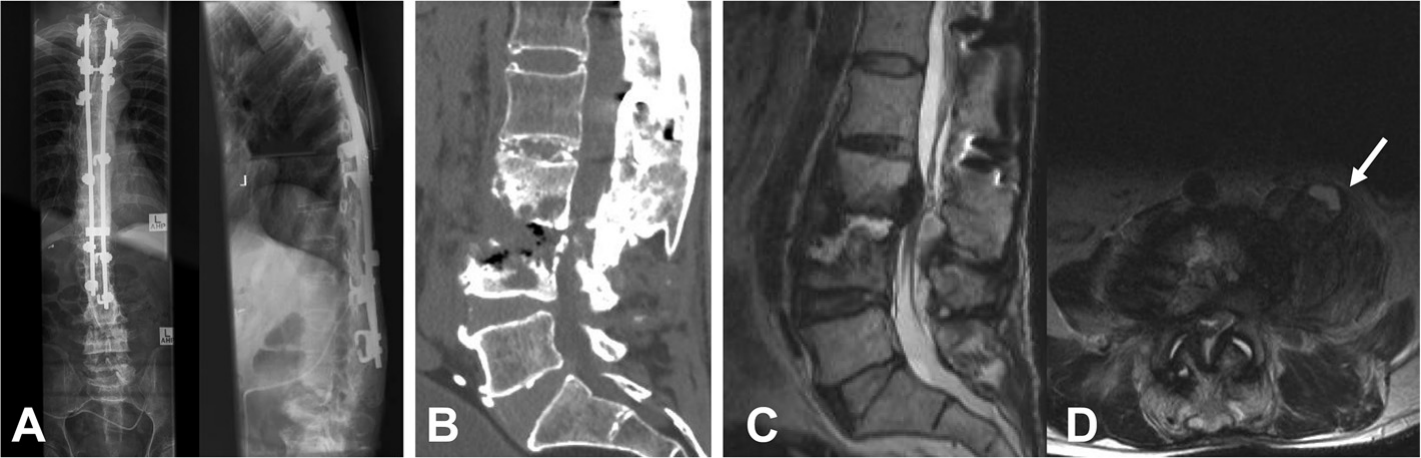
Plain radiographs, computed tomography and magnetic resonance imaging showing bone destruction and deformity consistent with Charcot spine below previous thoracolumbar instrumented fusion. (A) Plain anteroposterior and lateral radiographs showing previous instrumented fusion with hooks and rods from T3 to L3. Bony destruction and deformity is evident distal to the previous fusion. Sagittal computed tomography scan (B) and T2-weighted magnetic resonance imaging (C) showing extensive endplate and osseous destruction at L3 and L4. (D) Axial T2-weighted magnetic resonance imaging showing abnormal paravertebral soft tissue and a complex paraspinal mass within the psoas muscle with fluid collections, areas of dystrophic ossification and nonspecific soft tissue inflammatory changes (arrow).
